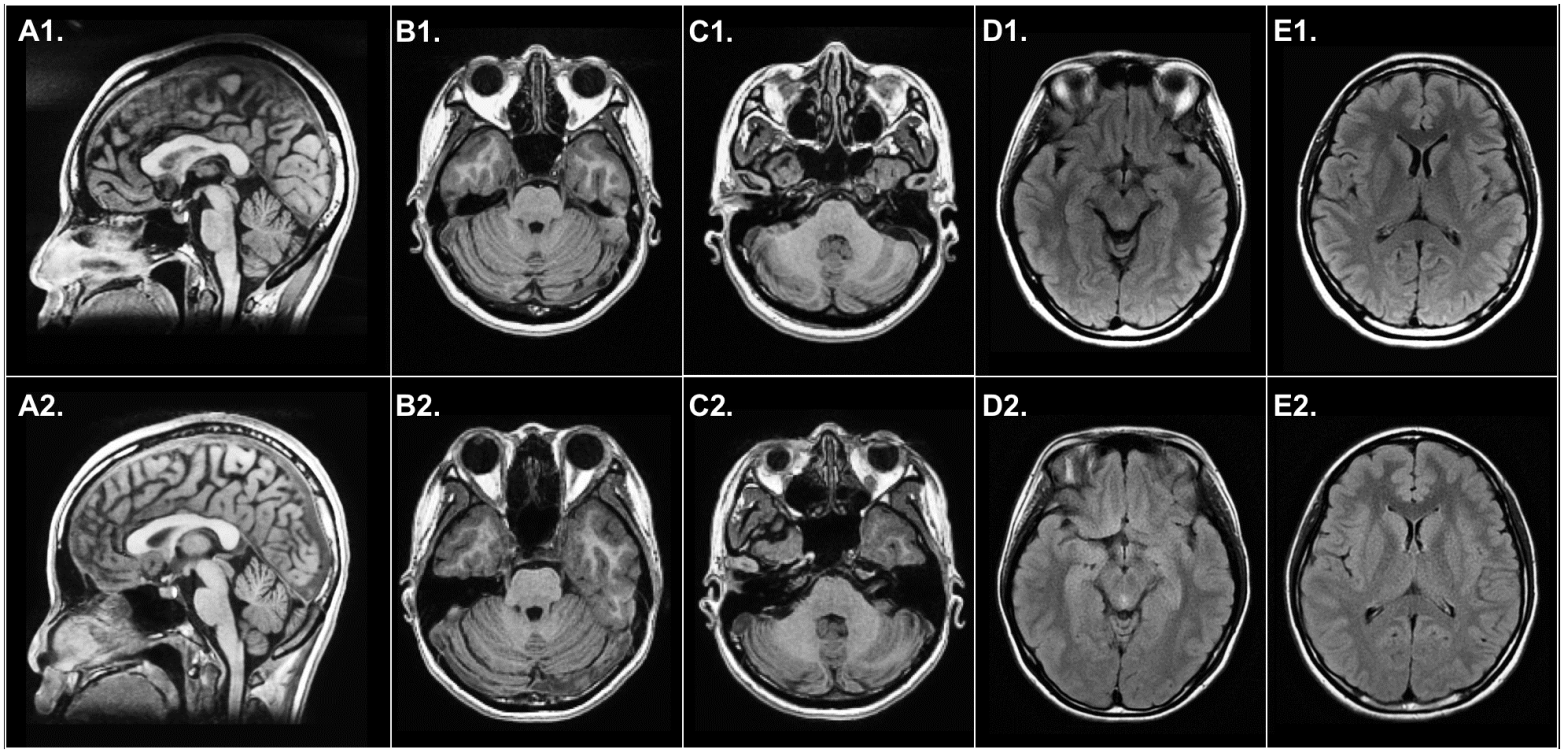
Brain MRI of the patients carrying *ITPR1* mutation. The neuroimages of II-3 are shown as A1-E1, and her daughter’s images (III-1) are A2-E2. The T1-weighted sagittal view images denote a mild atrophy of the anterior and posterior lobes of the cerebellar vermis (A1 and A2). The T1-weighted axial view images demonstrate a mild atrophy of the cerebellar hemispheres (B1-C1 and B2-C2). The sizes of the pons and cerebellar peduncles are within normal ranges. The fluid-attenuated inversion recovery (FLAIR) axial view image features normal cerebral cortex, basal ganglia and midbrain (D1-E1 and D2-E2).
